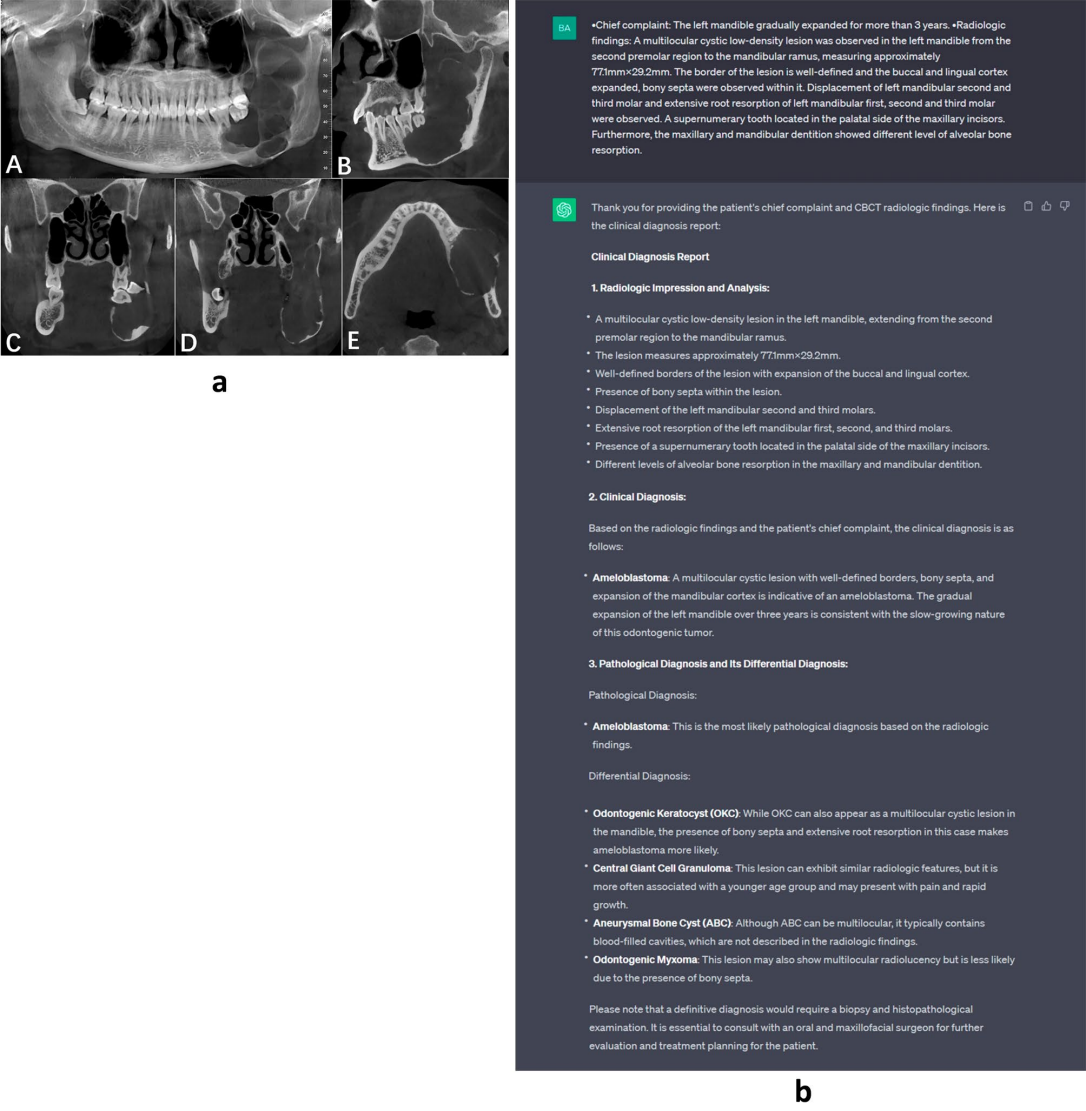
A The CBCT presentation of a mandibular ameloblastoma; B The input and output in ChatGPT
